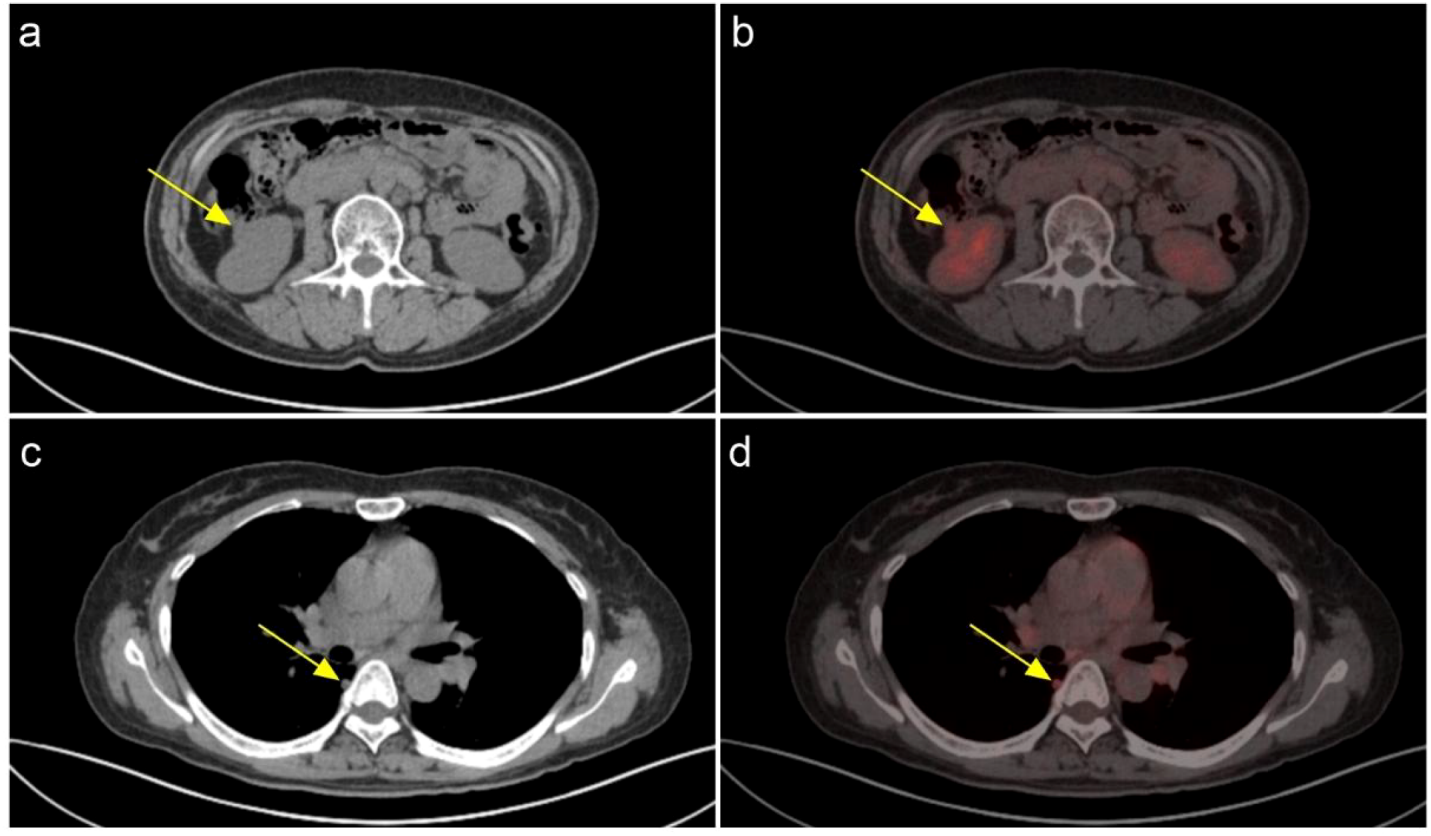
A soft tissue nodule is present in the lower pole cortex of the right kidney, with increased glucose metabolism. A neoplastic lesion cannot be ruled out. (A) CT (B) PET-CT; Multiple solid minute nodules are present in both lungs, with the largest one located paraspinally in the dorsal segment of the lower lobe of the right lung. (C) CT (D) PET-CT.
